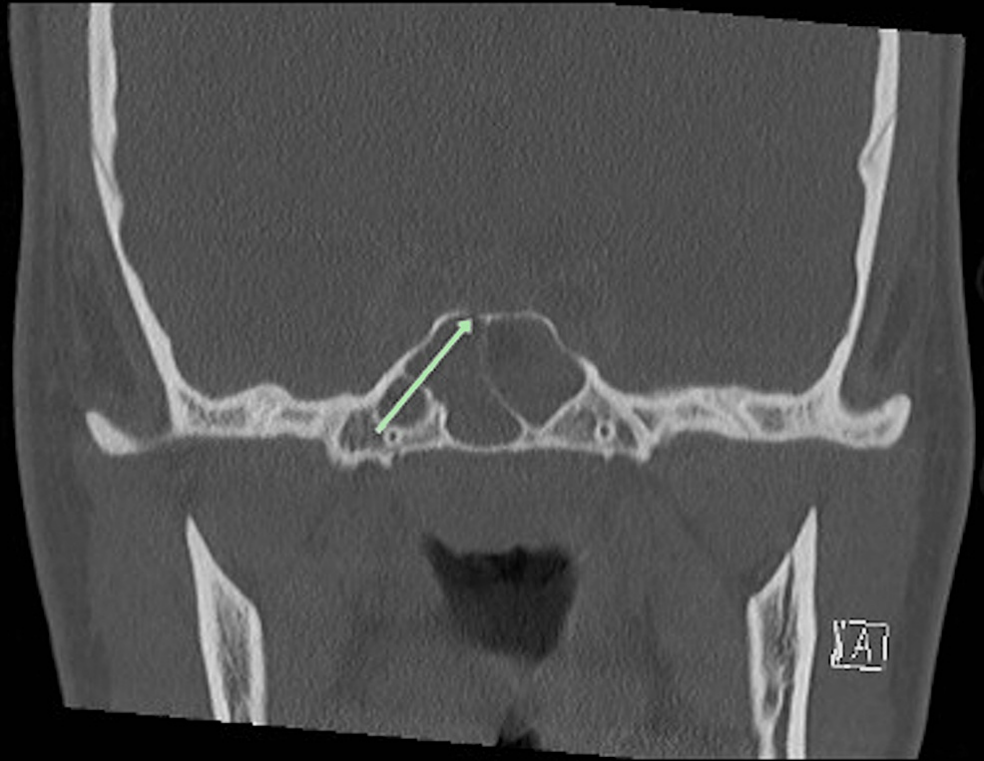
CT of the sinus with contrast, coronal cut, sphenoid sinus disease with bony dehiscence along superior right sphenoid sinus (arrow) CT, computed tomography.
